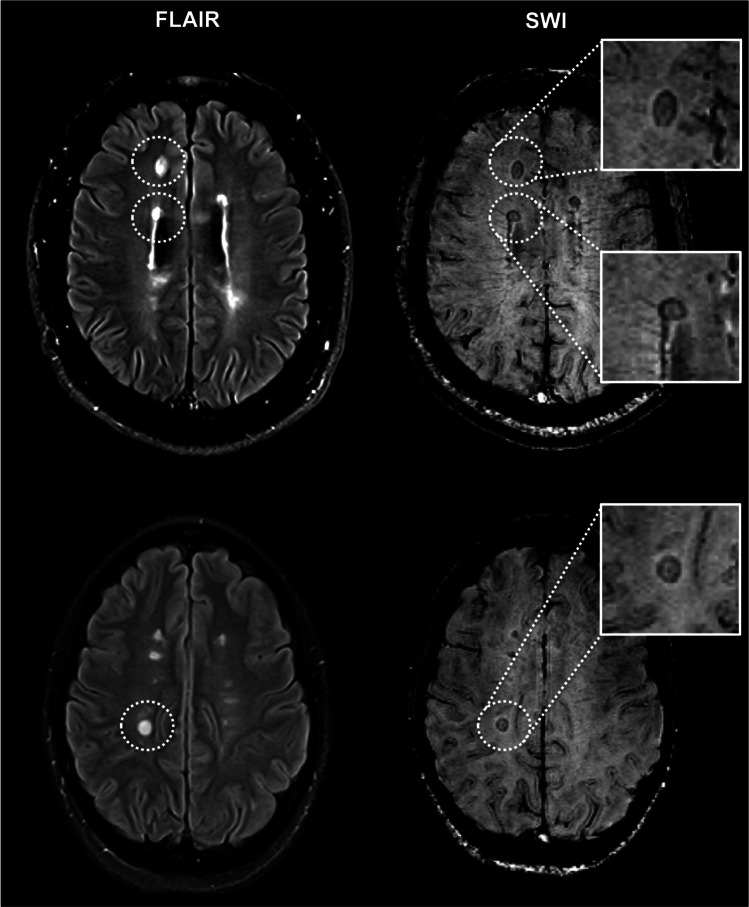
Representative examples of iron rim lesions (IRLs). Fluid-attenuated inversion recovery (FLAIR; left) and susceptibility-weighted images (SWI; right) demonstrate multiple IRLs (encircled and magnification)
